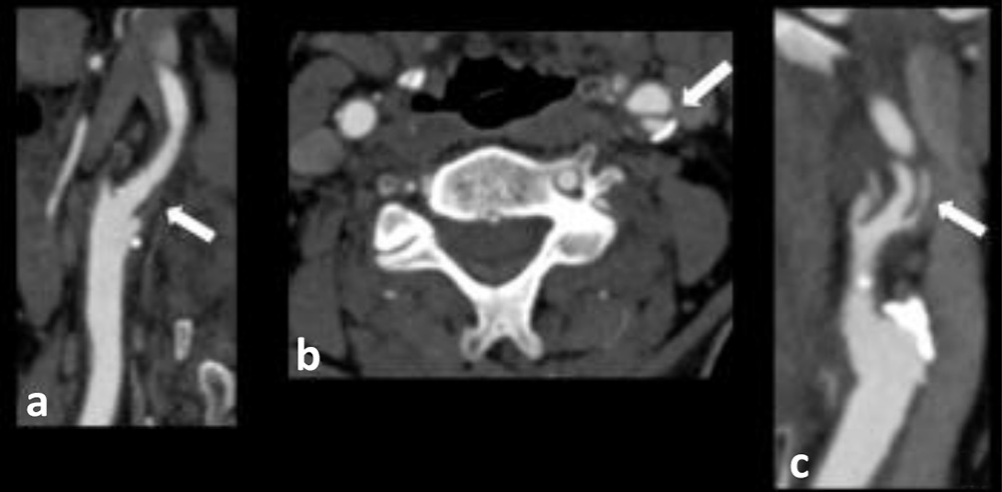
85-year-old male patient who presented with acute right-sided weakness. (a) Sagittal, (b) axial and (c) coronal CTA images demonstrate a carotid web arising from the posterior wall of the proximal left ICA. CTA, CT angiogram; ICA, internal carotid artery.
